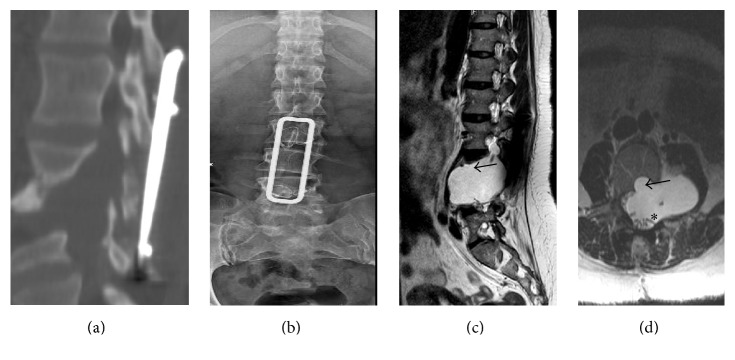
Postoperative CT and X-rays (a and b) showing L3 hemilaminectomy and L2–L5 stabilization with Hartshill rectangle and titanium wire. One-year follow-up after the first operation, sagittal and axial T2-weighted MRI images (c and d) disclosed a relapse of the operated pseudomeningocele at L3-L4 level with vertebral body remodelling (black arrow) and spinal roots displacement and convolution (asterisk).
